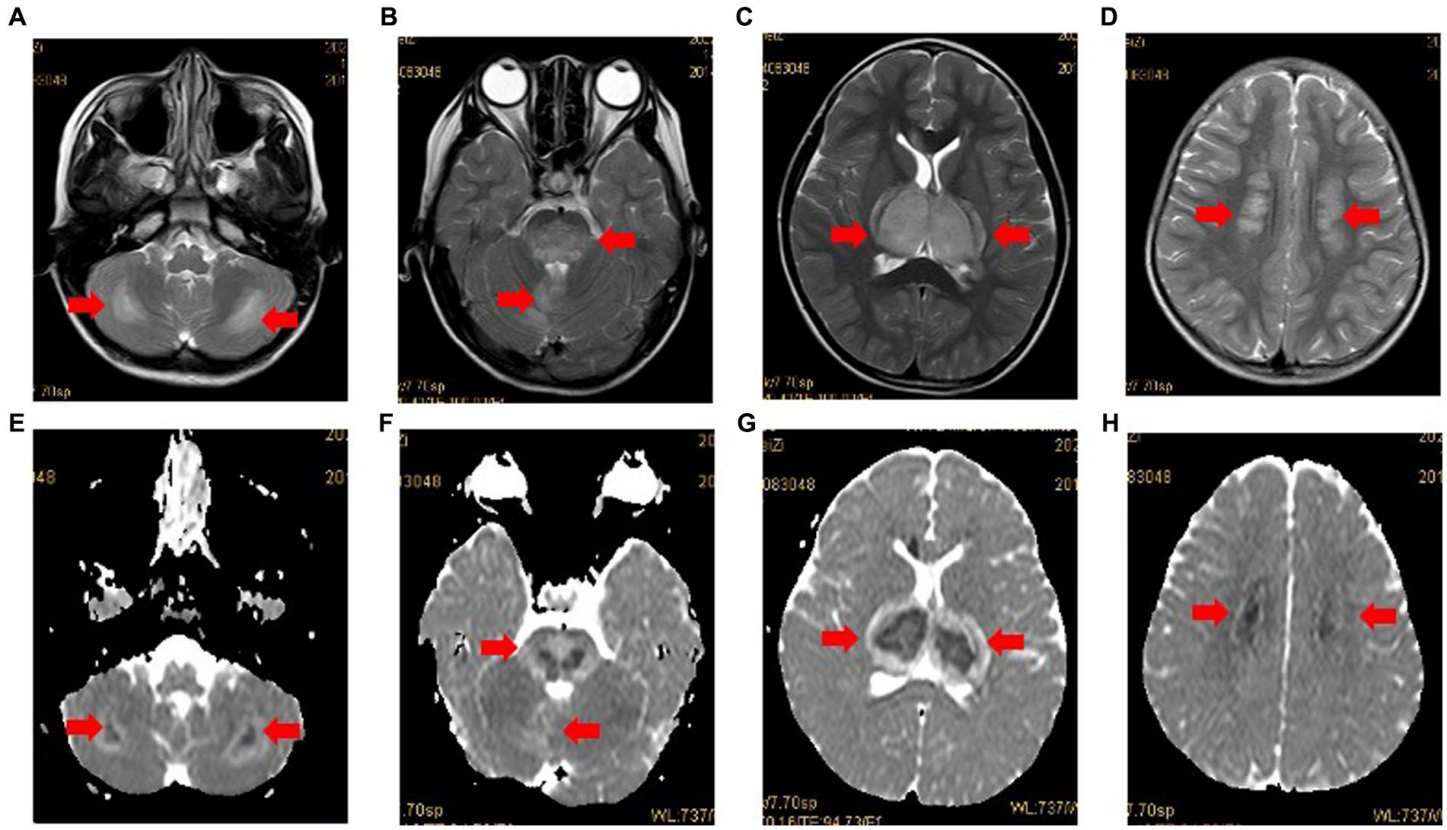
Case 3: MRI in case 3 with typical radiological findings of ANE. Axial T2-weighted images (A-D) showing bilateral symmetrical involvement of the cerebellum, brainstem, thalamus and cerebral white matter (arrows), respectively. The apparent diffusion coefficient (ADC) (E-H) presenting two gradient modes or there gradient modes.
